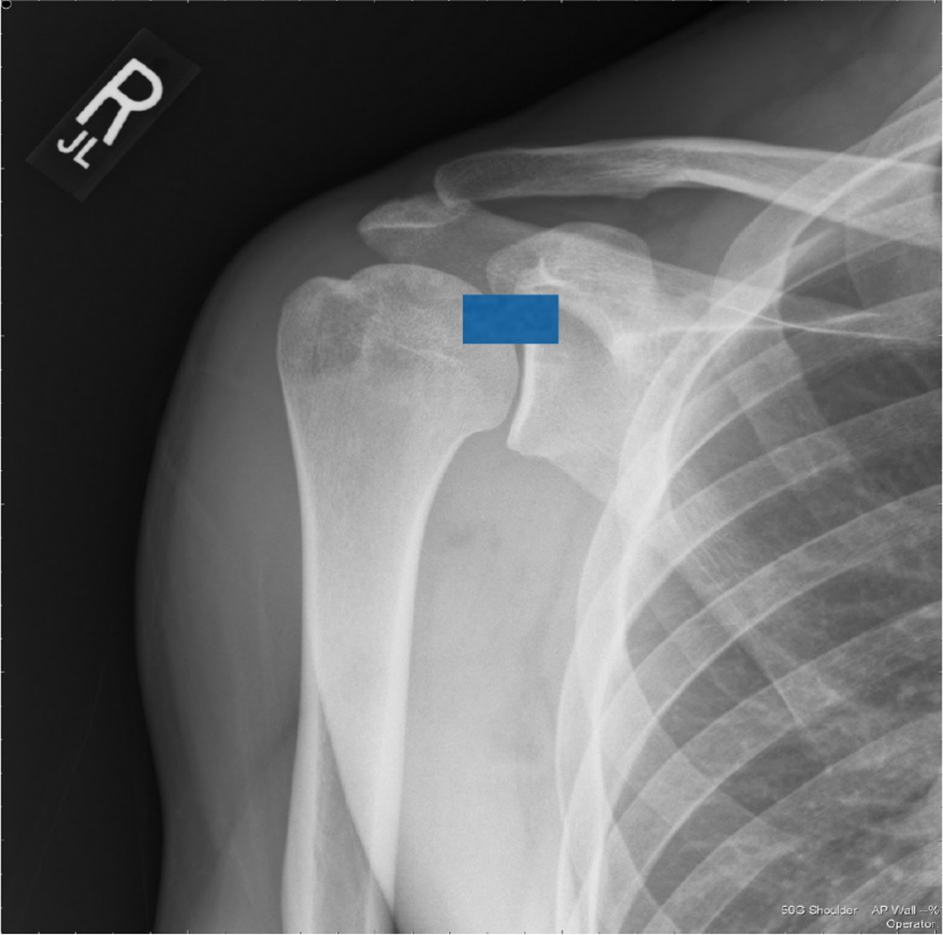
Position of the ultrasound probe at the level of the coracoid and humeral head.
